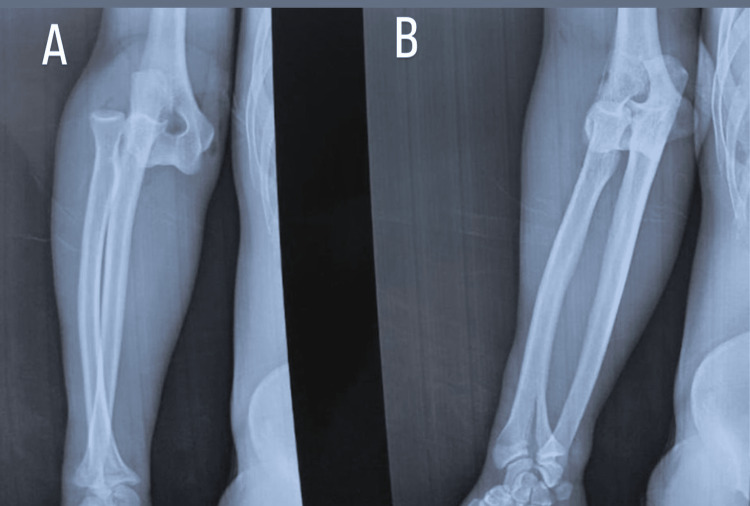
Elbow radiographs demonstrate posterior elbow dislocation before reduction, with no signs of bone lesions. (A) Anteroposterior (AP) view of elbow X-ray. (B) Lateral view of elbow X-ray
